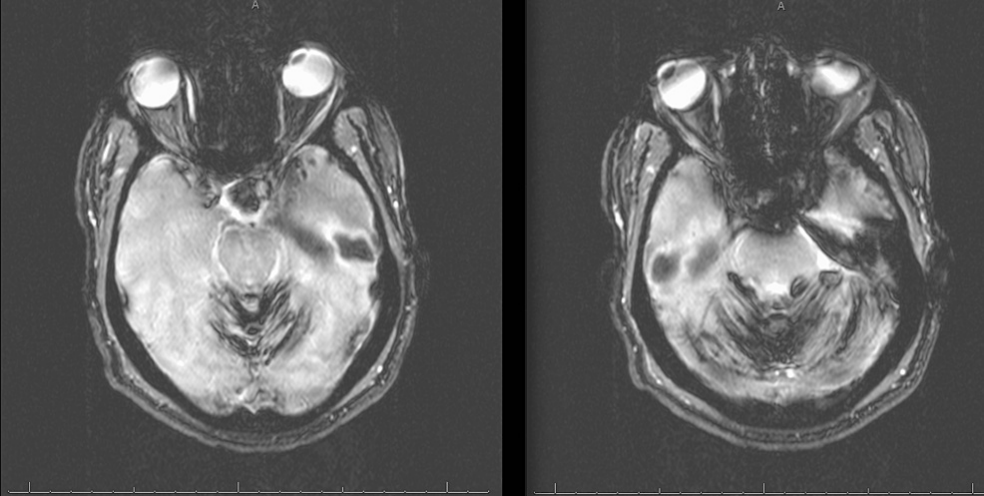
MRI with intravenous contrast of the brain: diffuse hemosiderin deposits in the cerebellar hemispheres bilaterally suspicious for superficial siderosis and hypointensities on plial surfaces.
